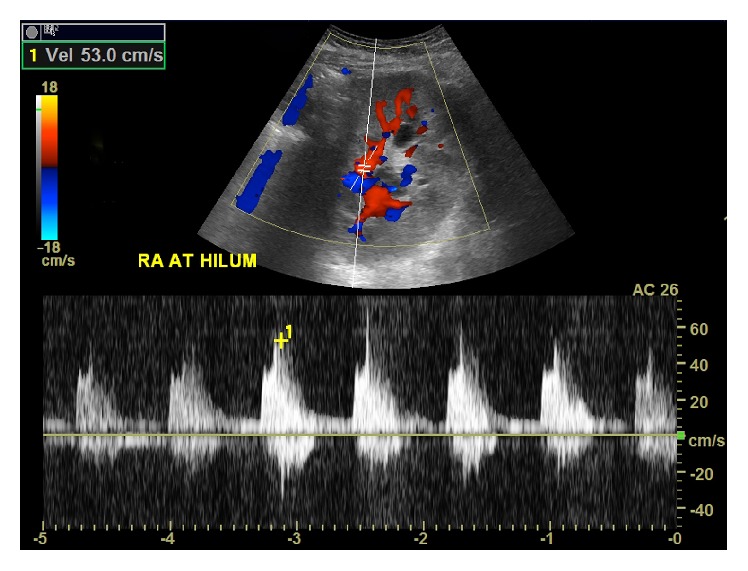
Doppler ultrasound performed on post-op day 1 after surgical detorsion demonstrated normal arterial blood flow with residual mild hydronephrosis and persistent enlargement of the renal allograft.
